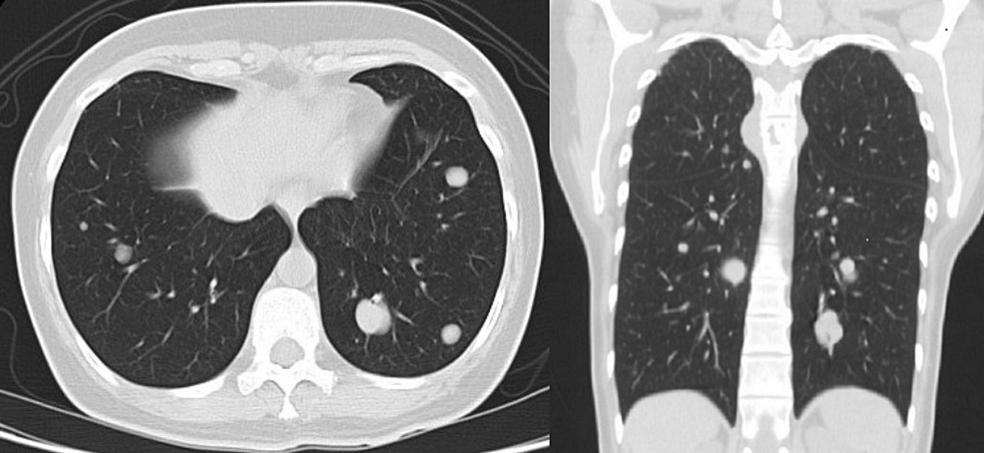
Chest CT with transverse and coronal slices demonstrating multiple heterogeneous nodules
